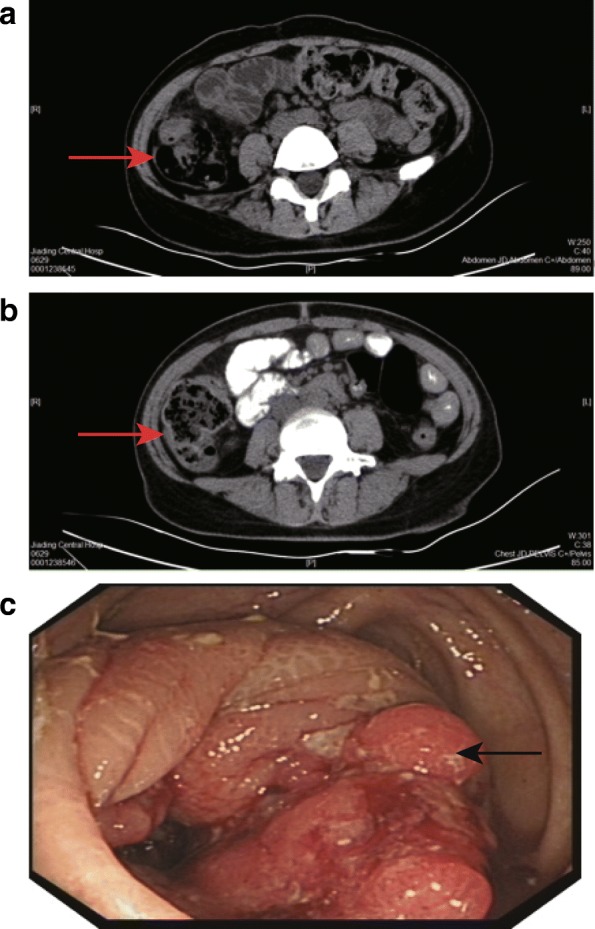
CT scan and colonoscopy showed wall thickening and a space-occupying lesion in the colon of the patient. a, upper abdominal CT scan showed wall thickening (red arrow) in the ascending colon; b, lower abdominal enhanced CT scan revealed wall thickening (red arrow) in the proximal ascending colon, distal cecum and ileum; c, a colonoscopy displayed a space-occupying lesion (black arrow) in the ascending colon. CT, computed tomography
